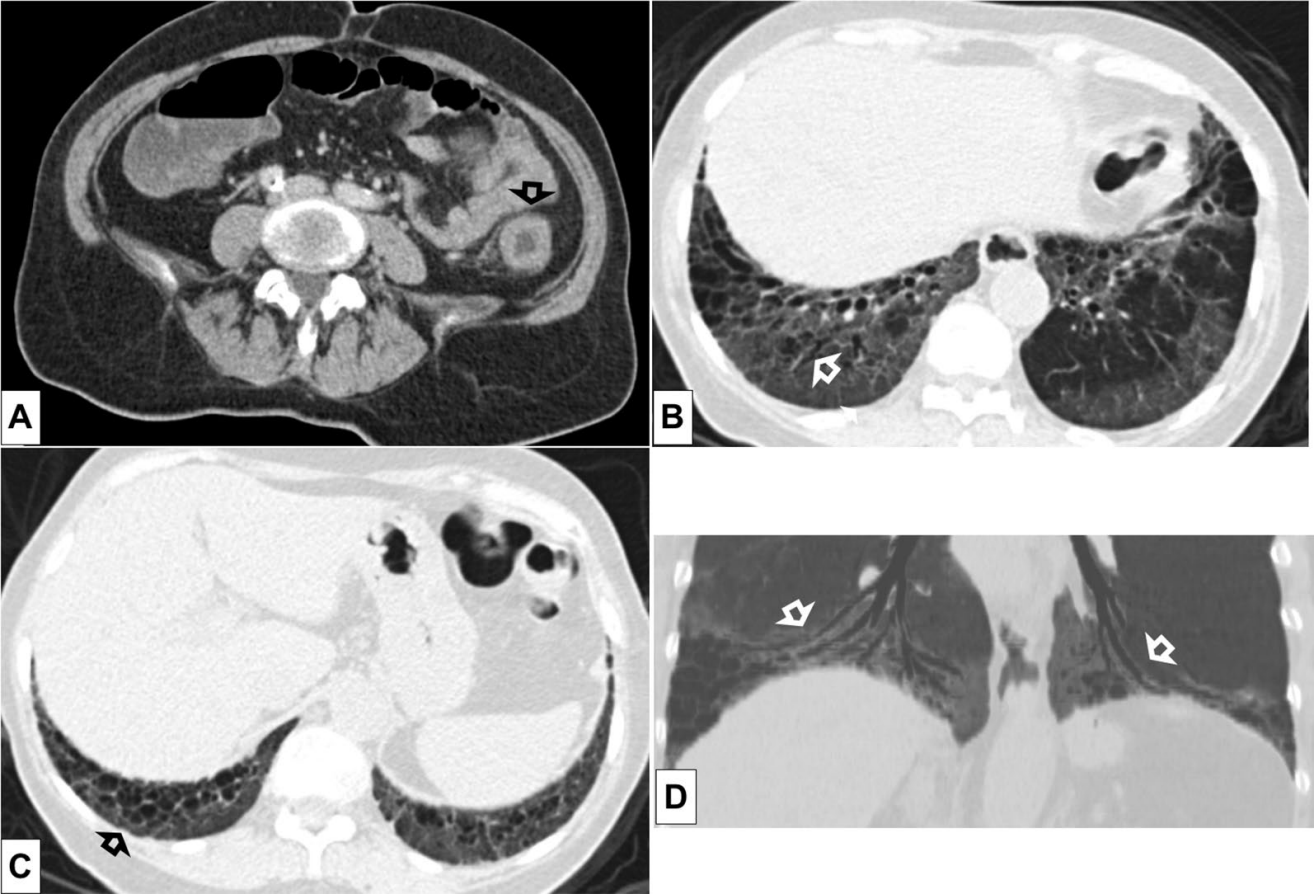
a–d. Axial (a) CT image of the abdomen with contrast (venous phase) of a 72-year-old gentleman shows wall thickening of the descending colon (*black open arrow*). Parenchymal window reconstructed axial CT images (b, c slice thickness of 2 mm) of the abdomen show right lower lobe traction bronchiectasis (*white open arrow*) and cystic airspaces with thick fibrous walls, suggestive of honeycombing (*black open arrow*). Minimum intensity projection (MinIP) reconstruction coronal image (d) shows lower-lobes traction bronchiectasis (*white open arrows*)
